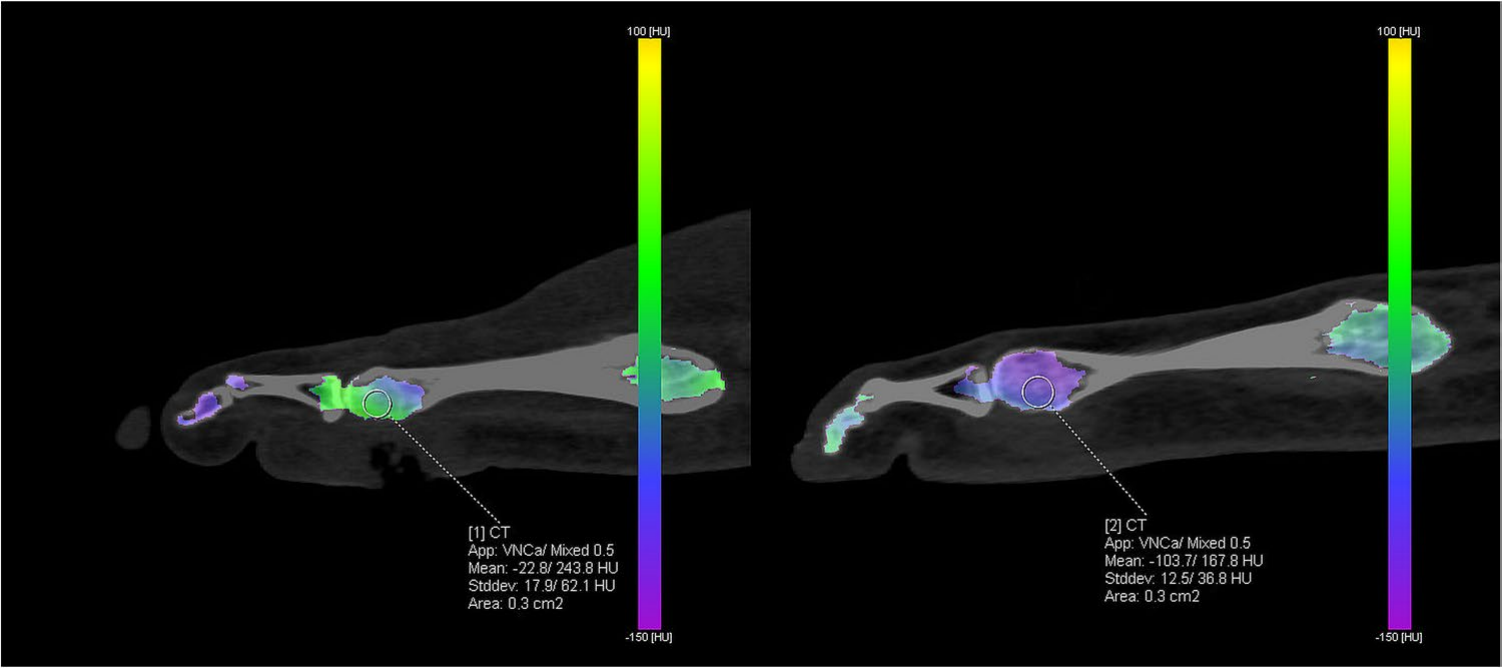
Measurement of CT values in the fifth metatarsal head on the ulcer side (left) and the reference side (right)
